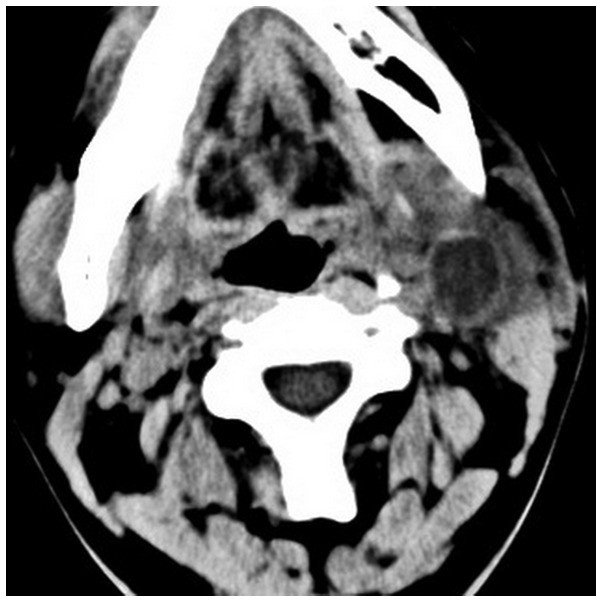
Type II BCC with infection. Axial plain CT scan revealed a left, poorly circumscribed mass with thickened walls in the neck. The density of the sternomastoid muscle and submandibular gland was found to be decreased and the adjacent fat planes were obscured. BCC, branchial cleft cyst; CT, computerized tomography.
